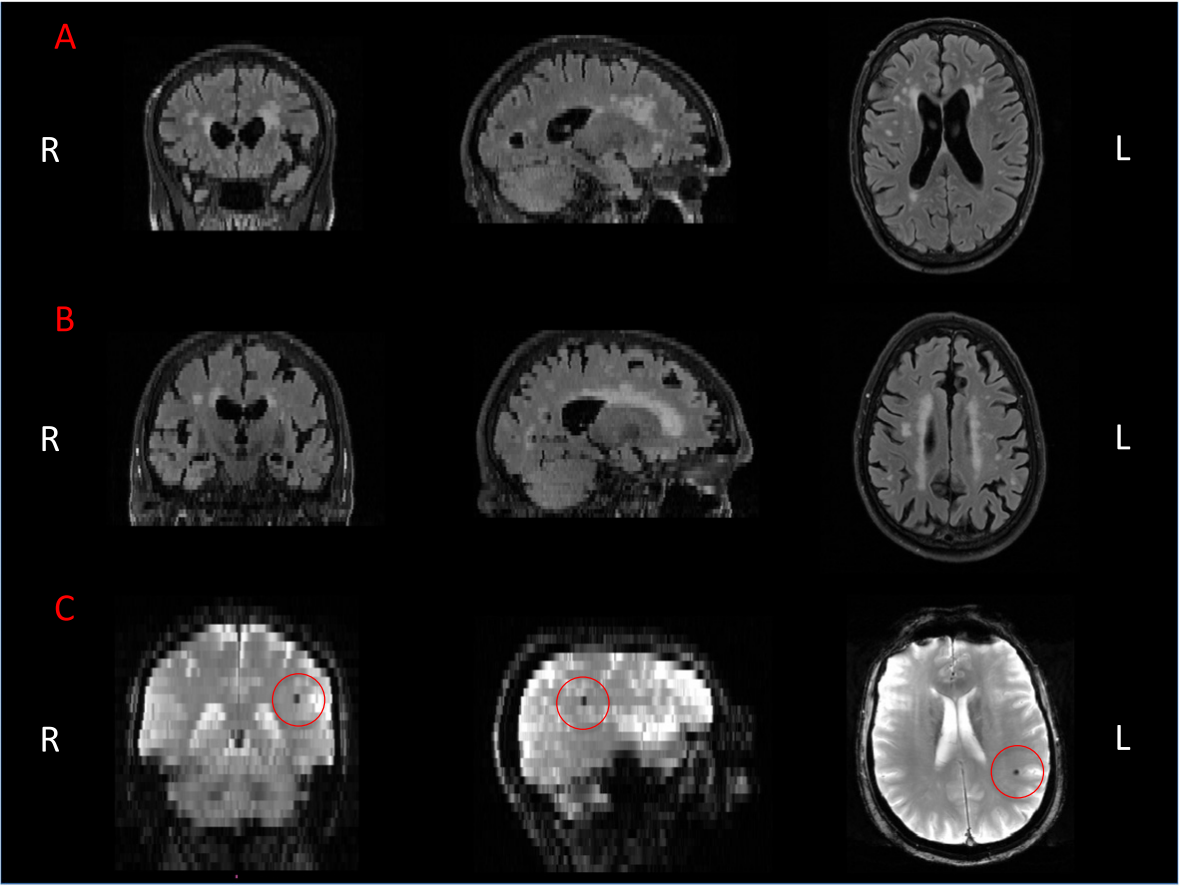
FLAIR and T2* images. (A) periventricular hyperintensities (PWH) and (B) deep white matter (DWM) lesions on FLAIR. (C) T2* image showing microbleed. R and L define the right and the left hemisphere, respectively.
